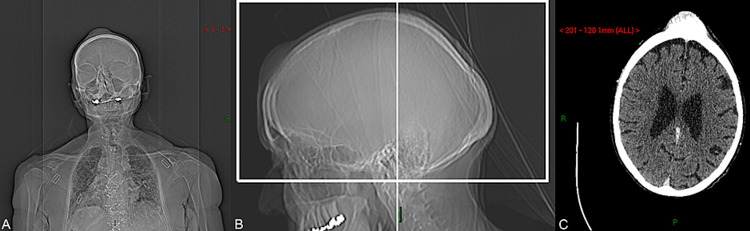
CT scan of patient in coronal (A), sagittal (B) and axial (C) views, respectively.
